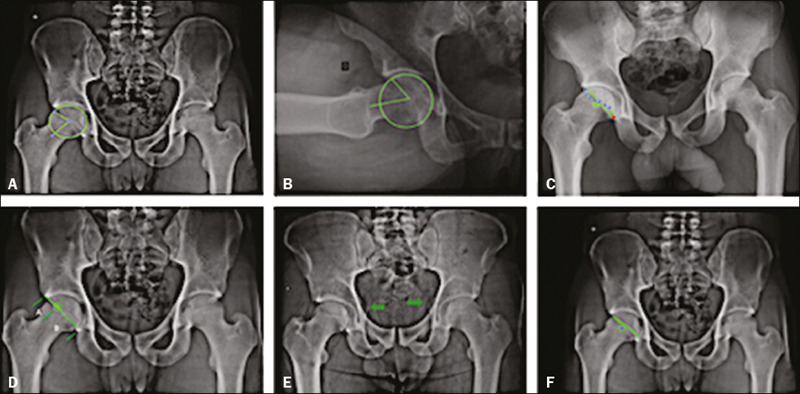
Alpha angle on anteroposterior X-ray (A); alpha angle on Ducroquet profile
